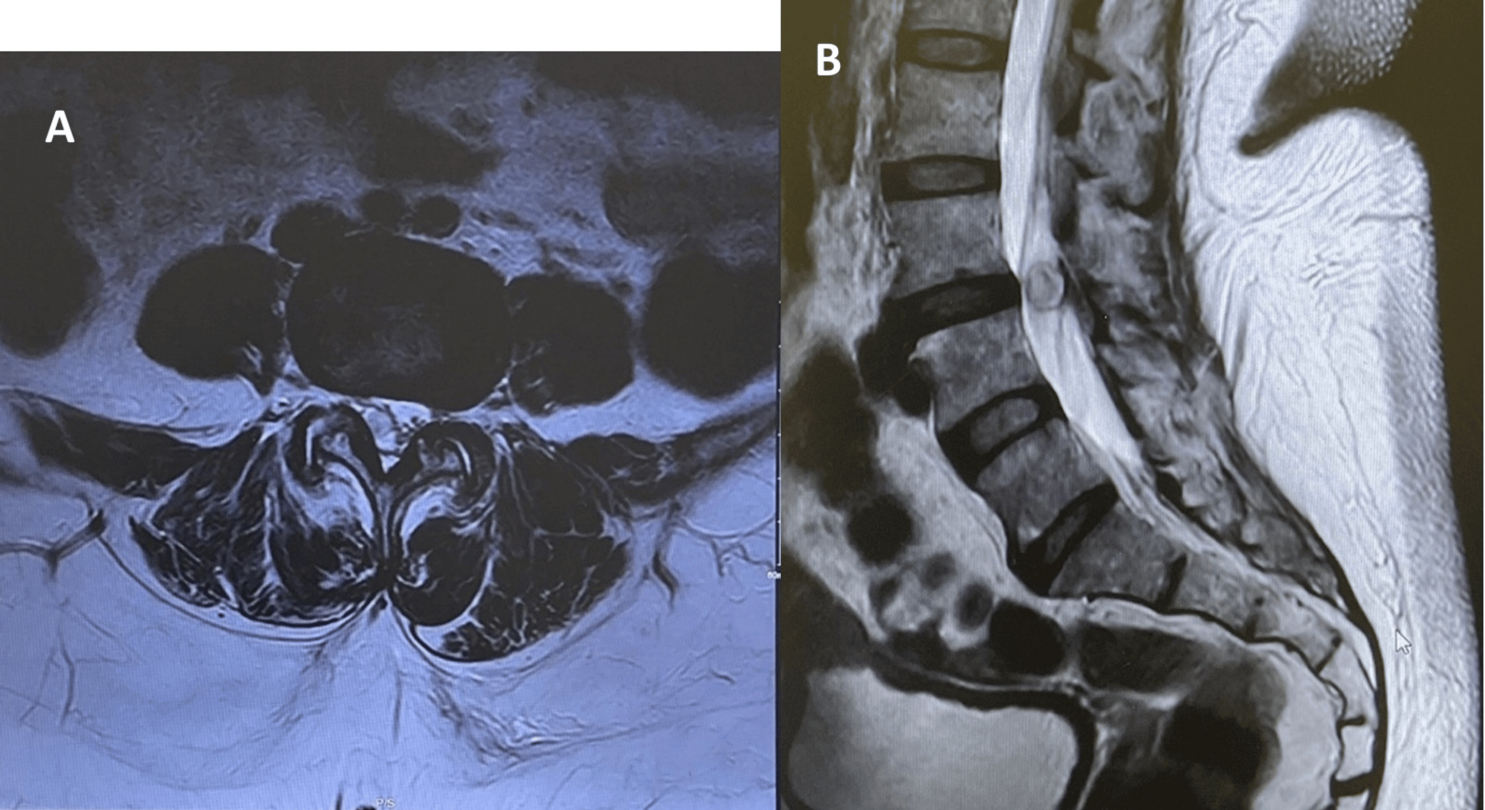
The patient's MRI findings Axial view (A) and sagittal view (B) show intraspinal lesions at L3–L4 with no disc bulging or herniation.
